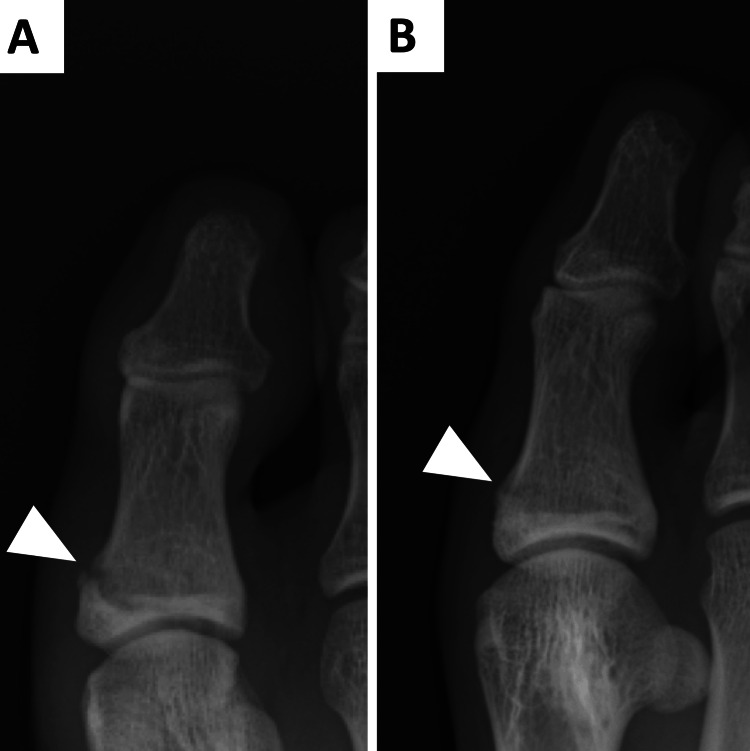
An X-ray six months after the initial diagnosis showed a non-union of the proximal phalanx of the great toe A: anteroposterior (AP) view; B: oblique view
